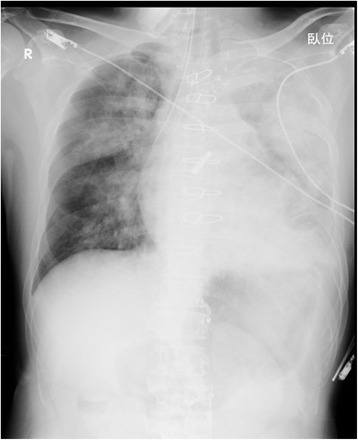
The chest X-ray shows diffuse consolidation of the left lung due to massive bleeding. A single-lumen endotracheal tube is placed in the right main bronchus
